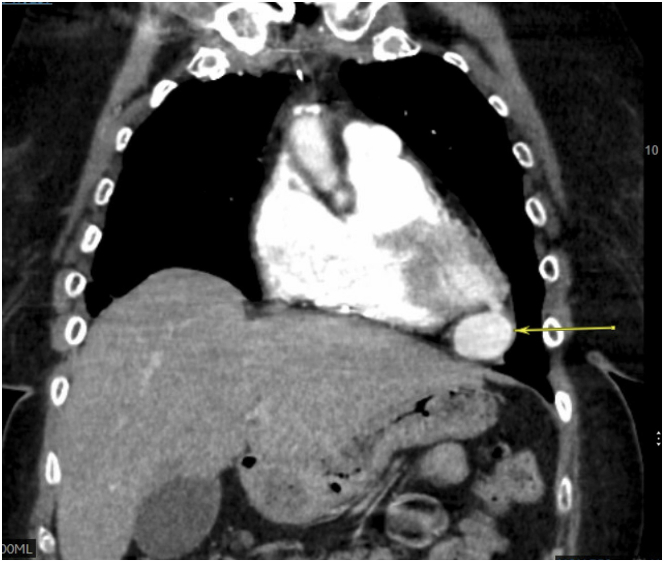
Computed Tomography Scan of the Chest Chest computed tomography with contrast enhancement demonstrates left ventricular pseudoaneurysm (arrow) at the apex of the left ventricle.
